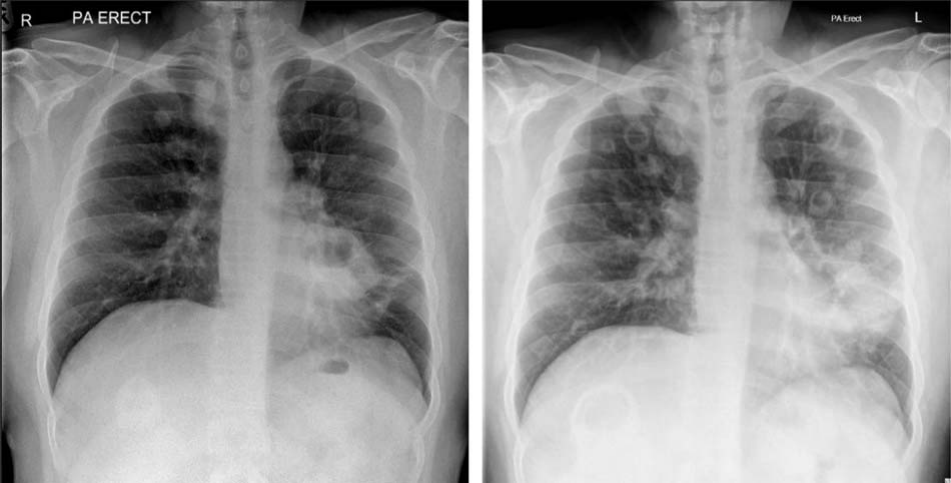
Chest radiographs showing progression of cavitating pulmonary lesions.
